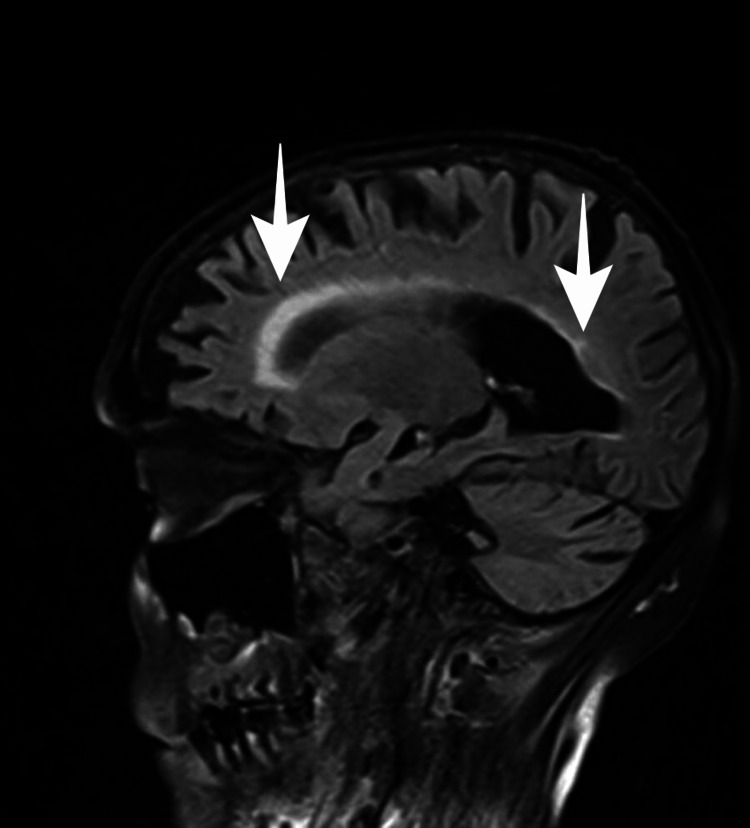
MRI sagittal T2 FLAIR images showing hyperintense lesions in the corpus callosum. FLAIR: fluid-attenuated inversion recovery
